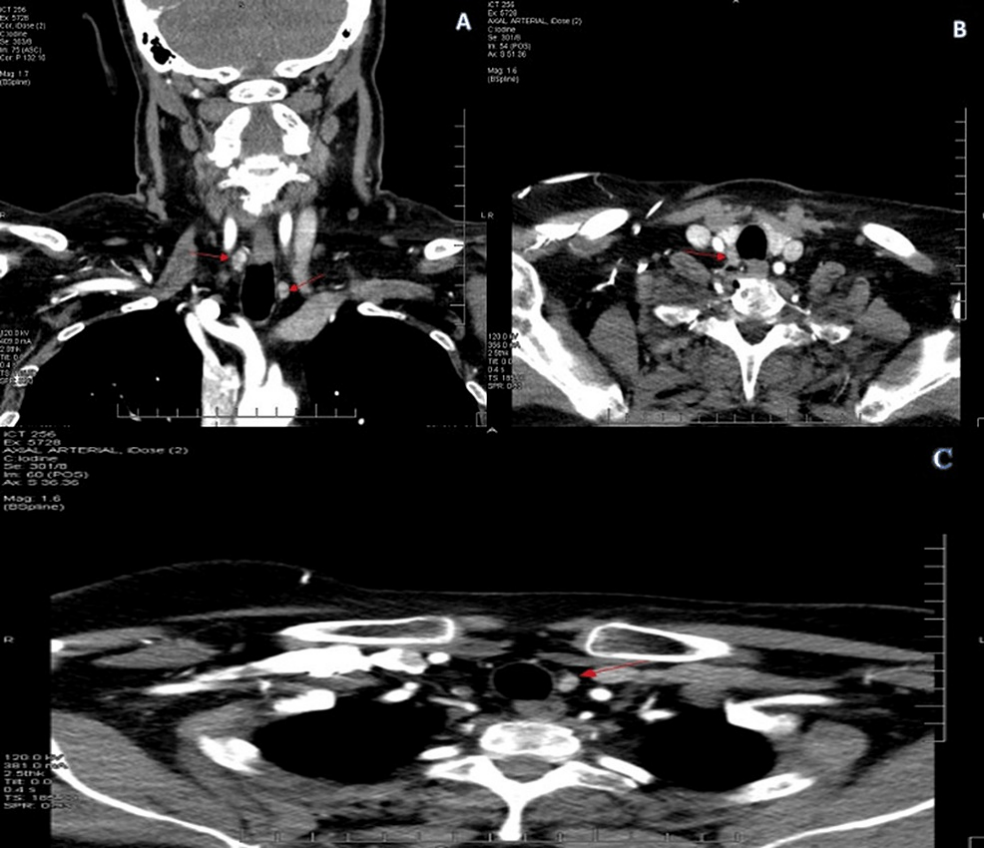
Four-dimensional computed tomography imaging was obtained prior to the patient’s bilateral neck exploration, demonstrating evidence of both the enlarged left inferior parathyroid nodule and right superior parathyroid nodule (red arrows). Panel A: Coronal view demonstrating the bilaterally enlarged parathyroid nodules (red arrows). Panel B: Axial view demonstrating the right-sided enlarged parathyroid nodule (red arrow). Panel C: Axial view demonstrating the left-sided enlarged parathyroid nodule (red arrow).
